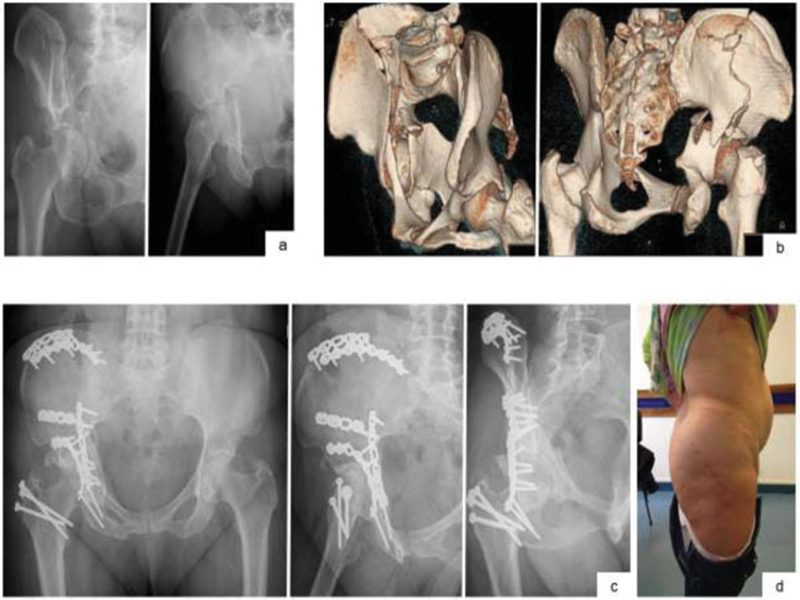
X-ray images at obturator oblique and oblique acetabular views, with typical spur sign on the first image (
